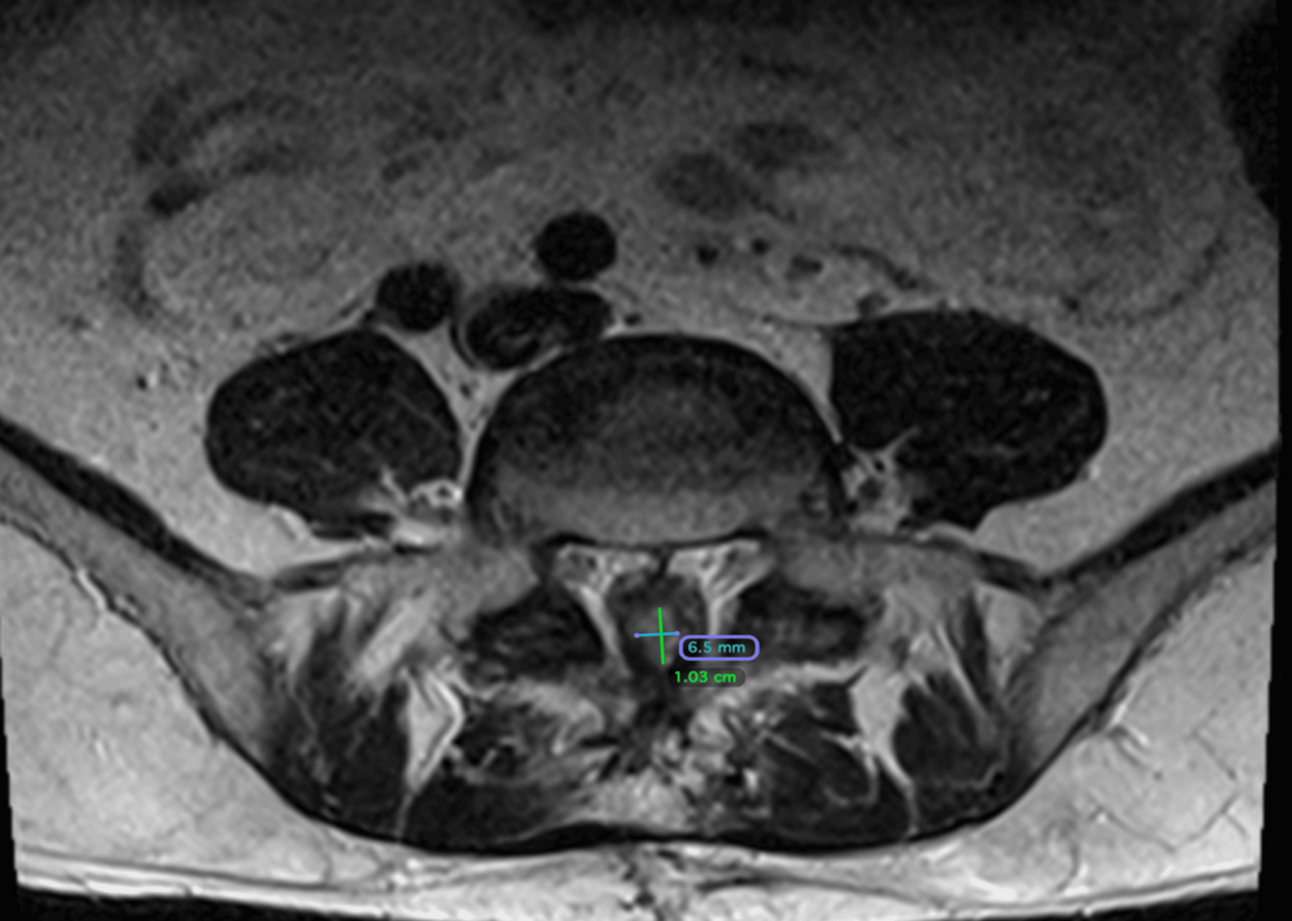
Axial image from an MRI L-spine dated June 20, 2024, approximately 13 months post cryoablation. The tumor measured 1.03 cm ant-post and 0.65 cm transversely.
